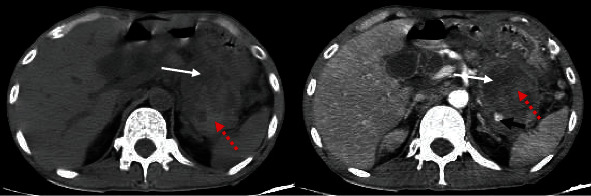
Axial contrast-enhanced computed tomographic images reveal a pseudoaneurysm at the pancreatic tail-omental bursa region adjacent to the gastric wall, which might be the cause of the bleeding (white arrow: pancreatic pseudocyst; black arrow: pseudoaneurysm lumen filling with contrast; red dashed arrow: thrombus).
